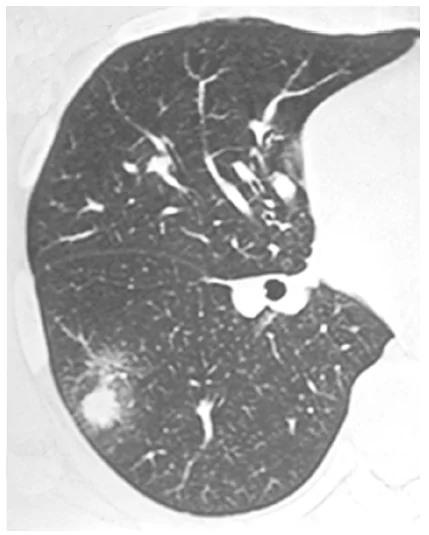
A CT scan with lung window setting at the level of the lower lobes, showing a nodule with soft tissue density in the right lower lobe, surrounded by a halo with ground-glass opacity (halo sign).
